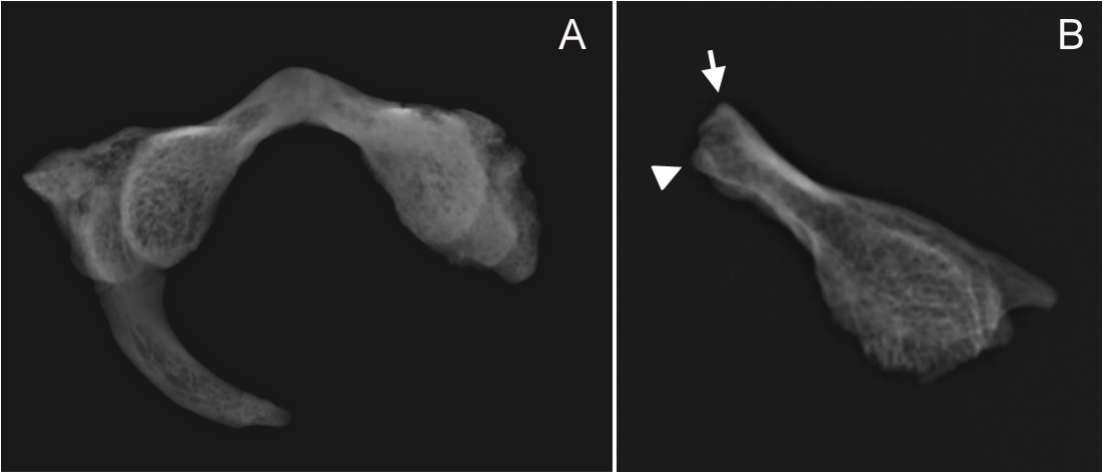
Radiographic images of SD-1643 and SD-1094. (A) Radiography of SD-1643, with continuity of the cortical bone through the irregularly-shaped tip of the left lamina. (B) Radiography of SD-1094, the arrow indicates the continuity of cortical bone at the anterior inferior corner of the midsagittal cleft (see Fig 2D and 2E), while the triangle indicates the continuity of cortical bone at the superior corner of the cleft (see Fig 2H and 2I). Lack of continuation of cortical bone through the entire cleft is due to post mortem breakage (see Fig 2F and 2G).
